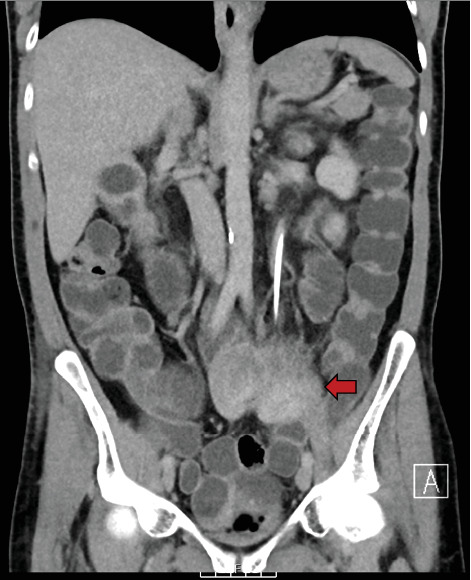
Initial CT urogram (coronal plane). Extravasation of contrast into the small bowel and fluid-filled small and large bowel.
